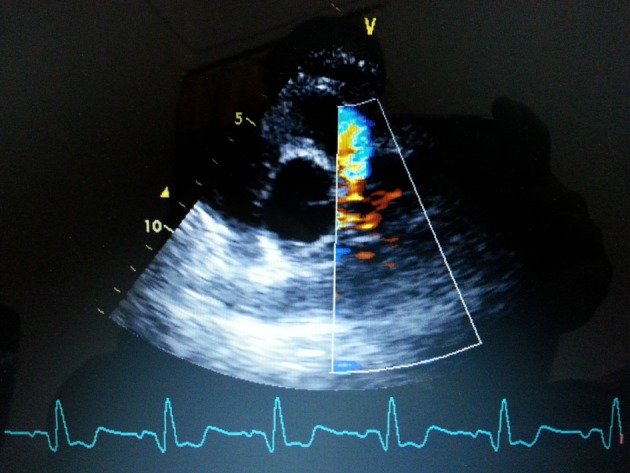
Transthoracic echo-parasternal short-axis view showing presence of continuous flow entering the pulmonary trunk.
